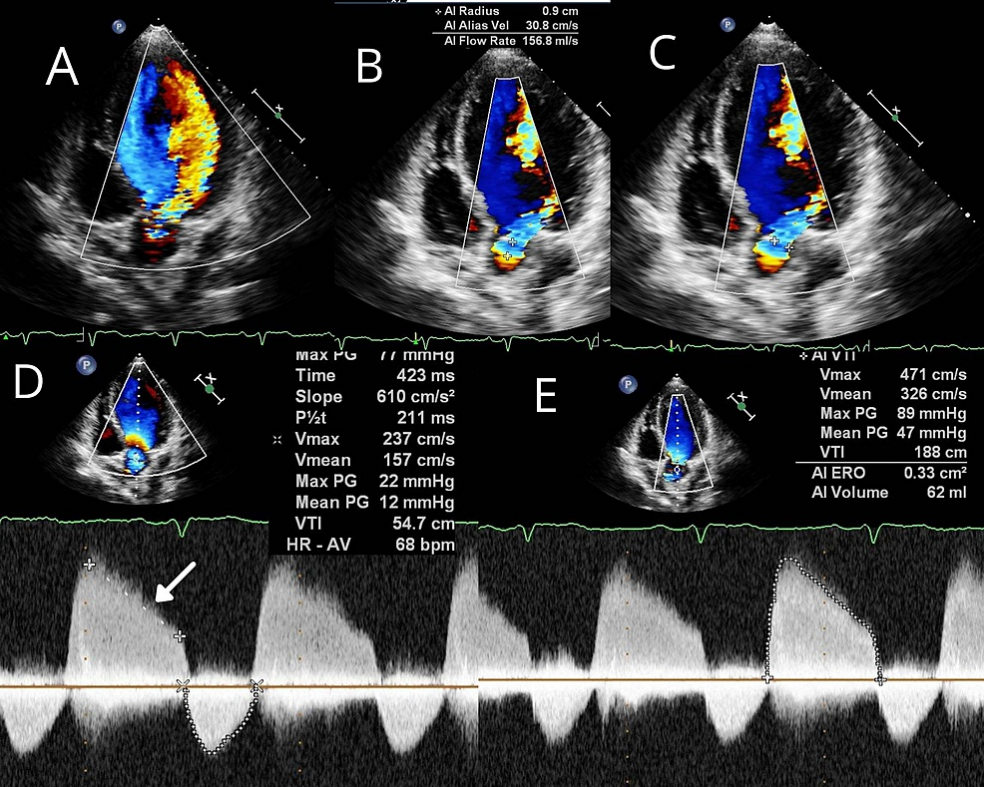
Transthoracic echocardiogram of aortic regurgitation (A) Five-chamber view showing eccentric aortic regurgitation. (B) Five-chamber view showing proximal isovelocity surface area of 0.9 cm. (C) Five-chamber view showing vena contracta 0.883 cm. (D) Five-chamber view with continuous-wave Doppler showing aortic deceleration slope 610 cm/s^2 ^and pressure half time 211 ms. (E) Five-chamber view with continuous-wave Doppler showing effective regurgitant orifice area of 0.33 cm^2^.
